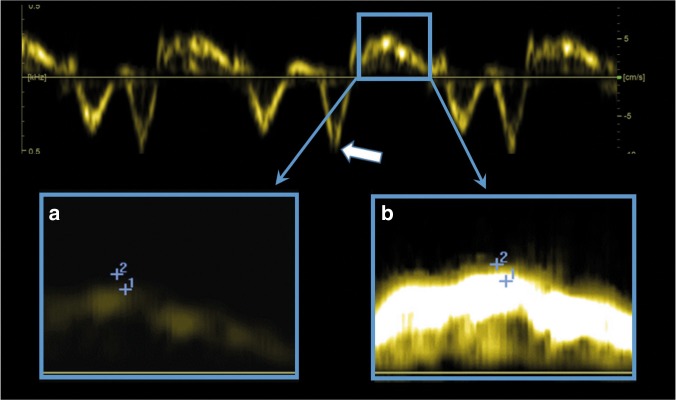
Pitfalls in image acquisition and analysis of pulsed-wave tissue Doppler images. The upper panel shows small breaks in the spectral curve when the ultrasound system records 2D images and pulsed-wave tissue Doppler spectral curve simultaneously. The a′ is false low in the second of the three heartbeats because the ultrasound system recorded the 2D image instead of the spectral curve at the time-point for the late diastolic peak (white arrow). The lower panel shows the systolic phase of one heart cycle with assessment of s′ velocity by correct (a) and by too high (b) gain setting. Measurement #1 is the correct velocity (5.1 cm/s) and measurement #2 is a false high velocity (6.1 cm/s). The only difference between the lower left and right panels is the gain setting during the off-line analysis
